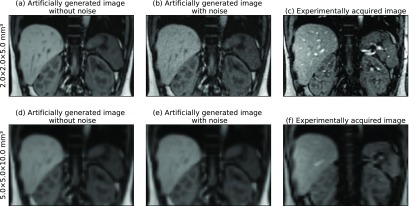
Side-by-side comparison of artificially created images (with and without added Rician noise) and experimentally acquired 2D cine MR images at (a)–(c) high and (d)–(f) low image resolution. Despite some differences in contrast, especially in blood or bony anatomy, the soft-tissue contrast and signal-to-noise ratio is comparable in artificial and real images.
